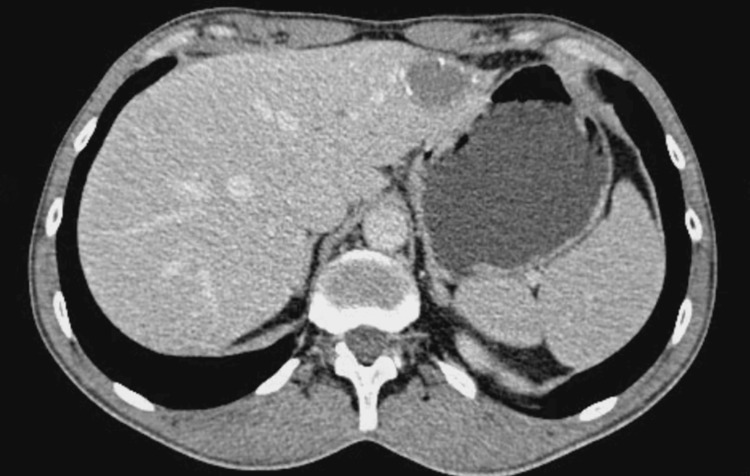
Contrast-enhanced CT demonstrating partial radiological response after nivolumab therapy Abdominal CT image showing a marked reduction in the size of the hepatic lesion in the left lobe (segments II–III) following systemic treatment with nivolumab, consistent with a sustained partial radiological response compared to baseline imaging.
